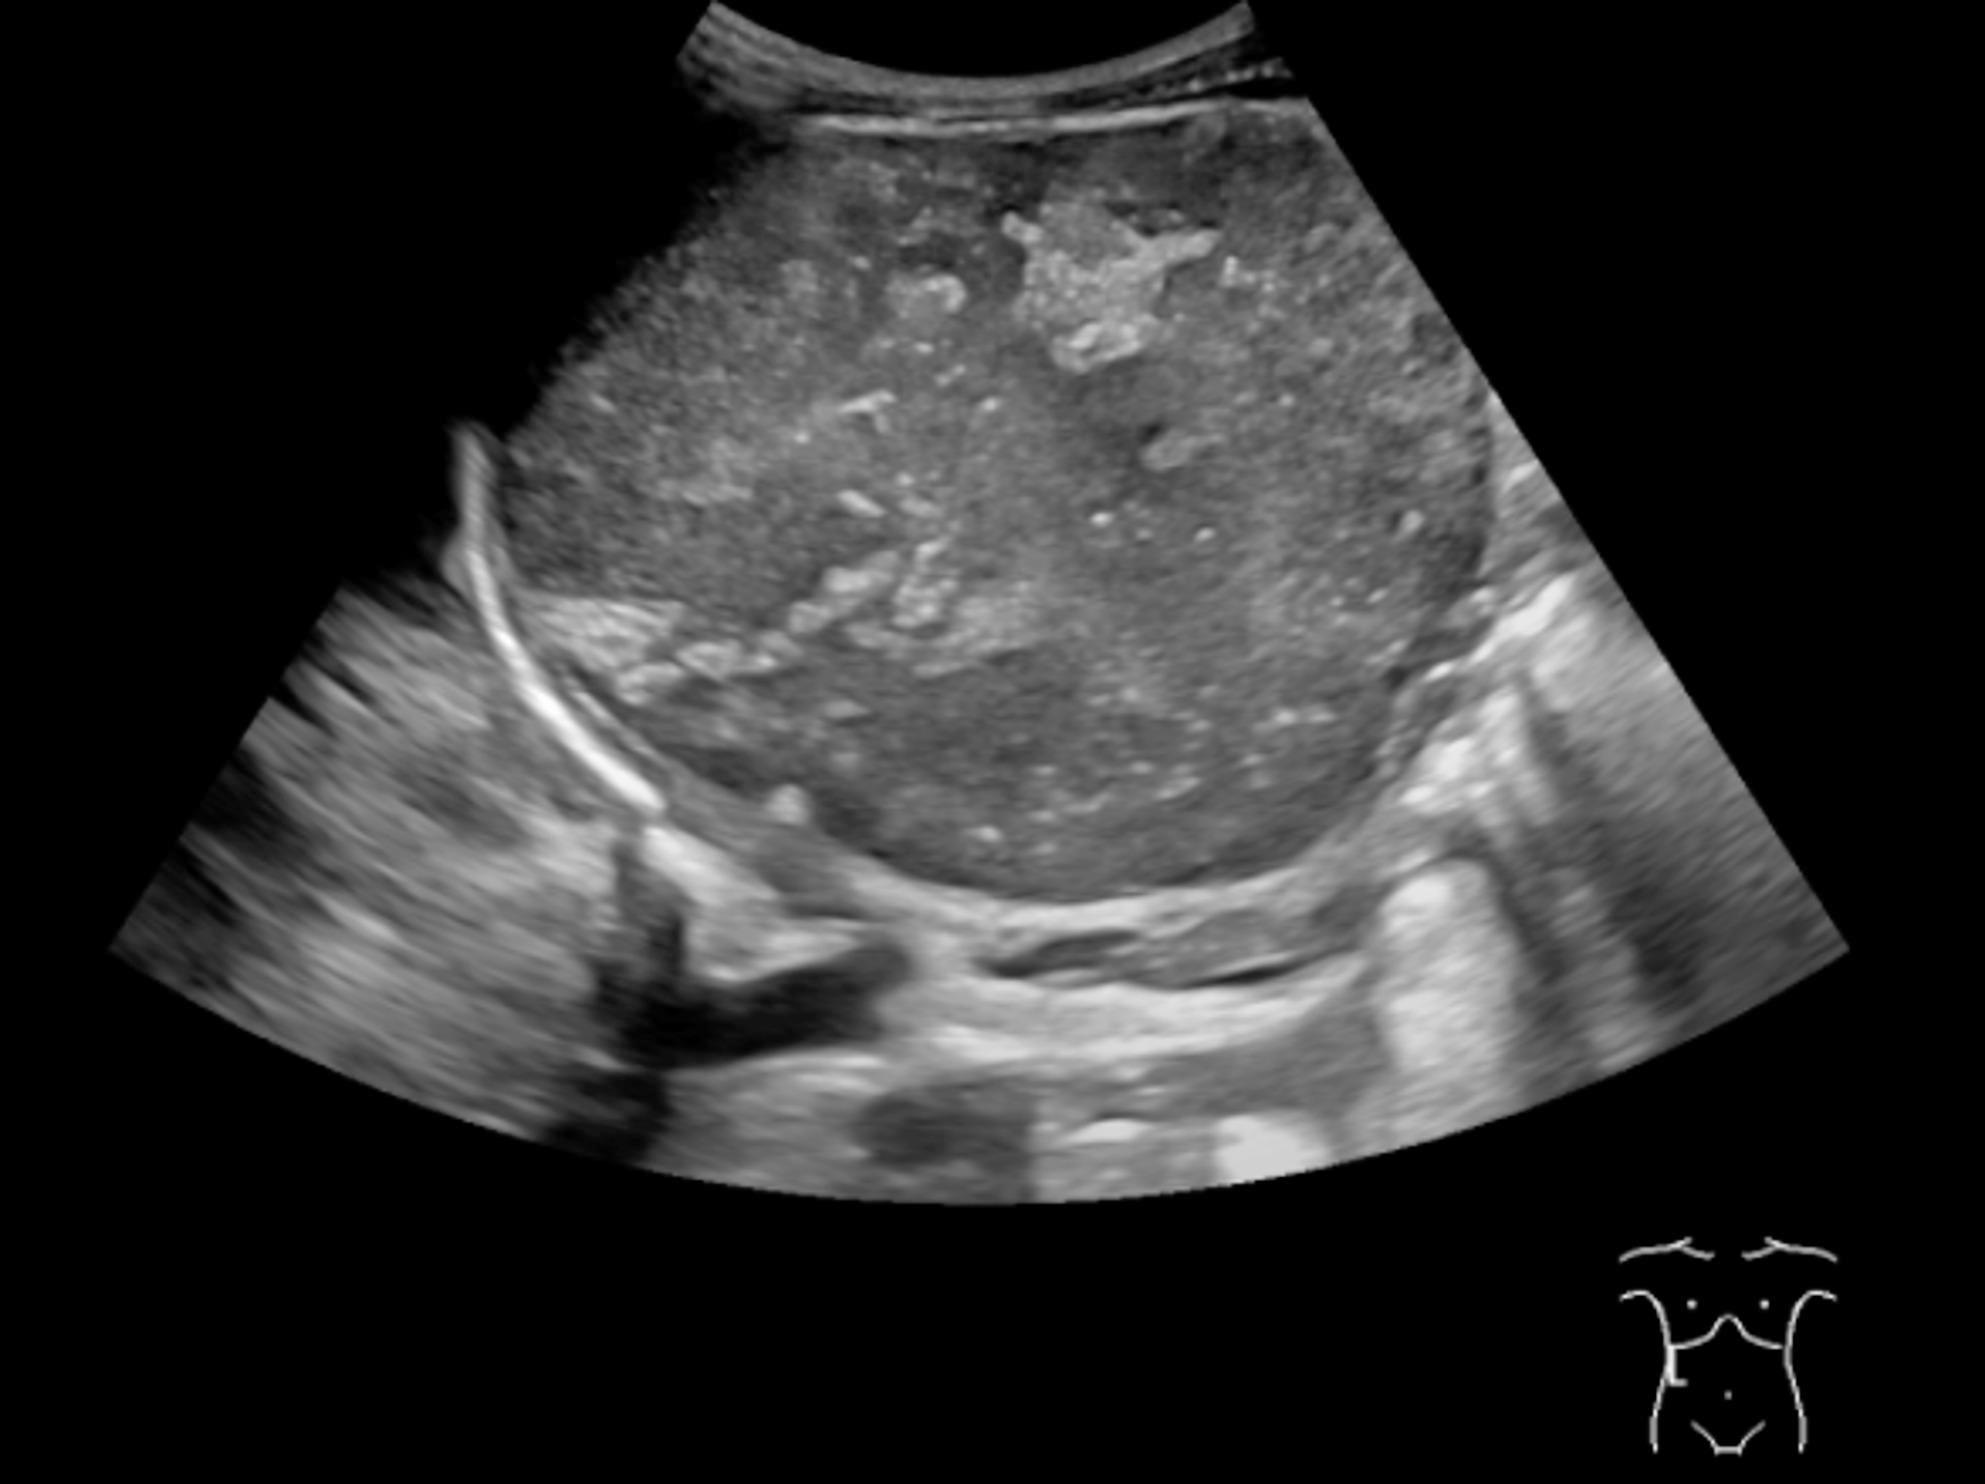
Axial T2-weighted MRI sequence of the abdomen, without contrast agent; slice thickness 3 mm
